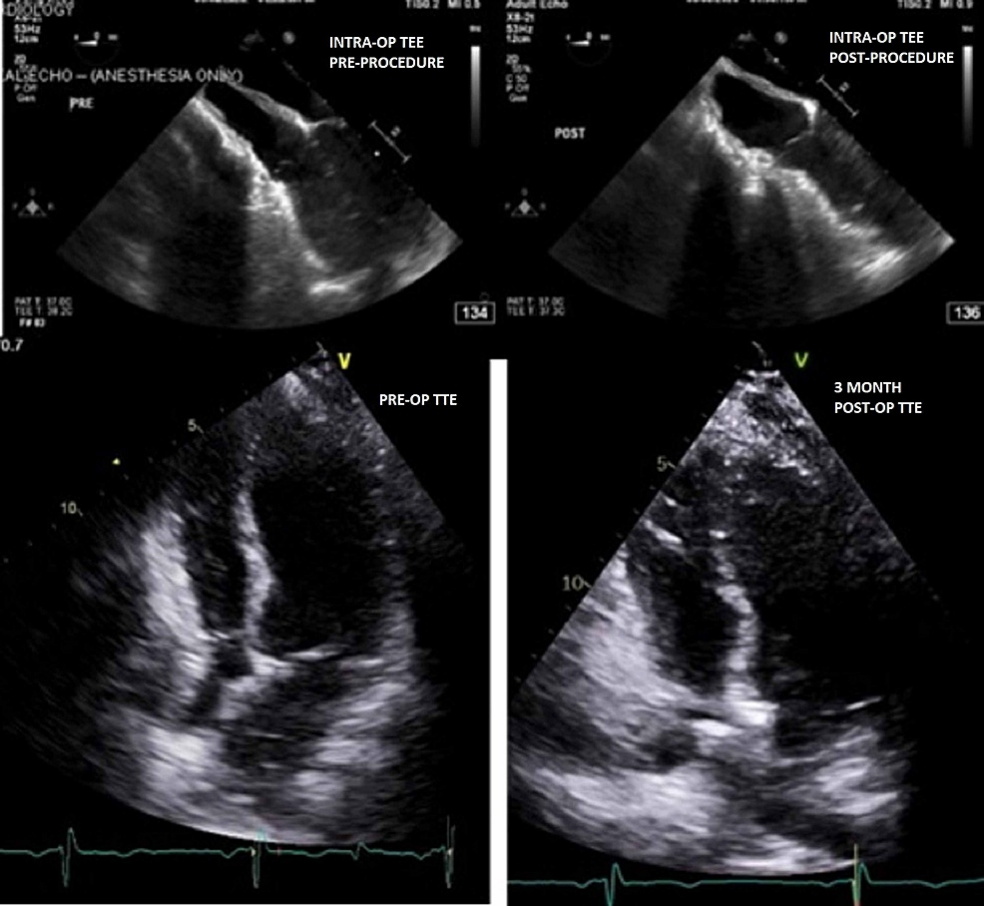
Intraoperative transesophageal echocardiography (top): Modified mid-esophageal 4-chamber view pre- (left) and post-procedure (right) Transthoracic echocardiography (bottom): Preoperative (left) and 3 months postoperative (right)
